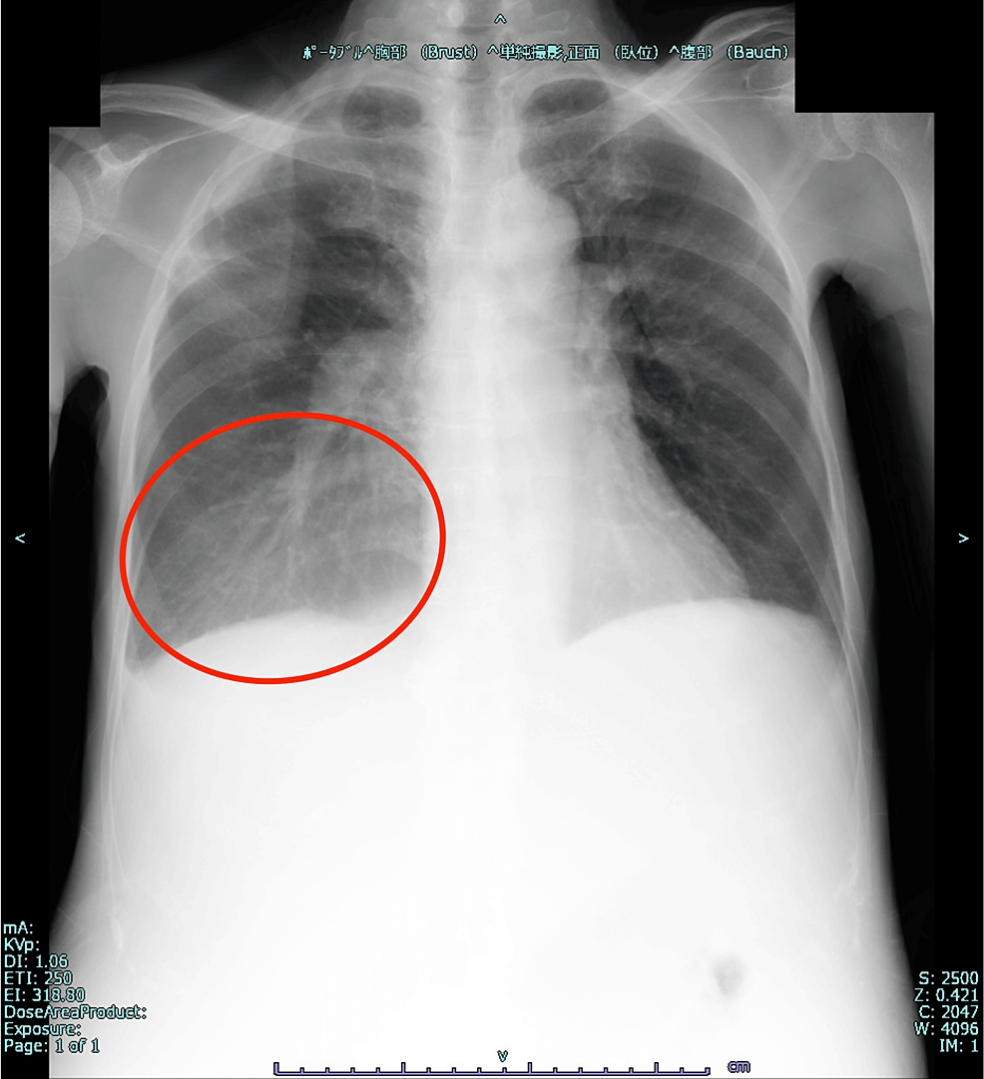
Chest radiograph (prone) Right pleural effusion (red round) and no evidence of pulmonary congestion or cardiac enlargement are observed.
